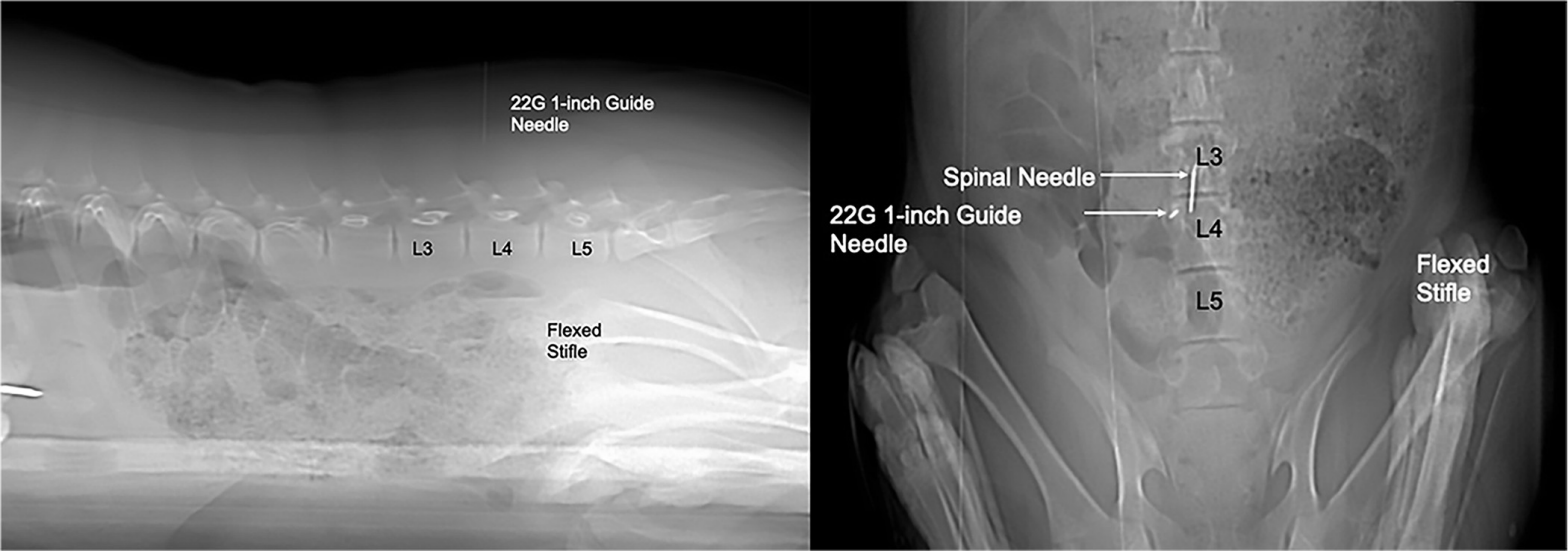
Sagittal and dorsal CT scout images showing guide needle and spinal needle placement. The guide needle is used to determine the site of insertion of the spinal needle on the skin. The needle is placed perpendicular to the long axis of the spine and immediately cranial to the border of the flexed stifle and directed perpendicularly. The spinal needle is placed into the vertebral canal immediately cranial to the guide needle and advanced until the tip meets the ventral surface of the spinal canal.
